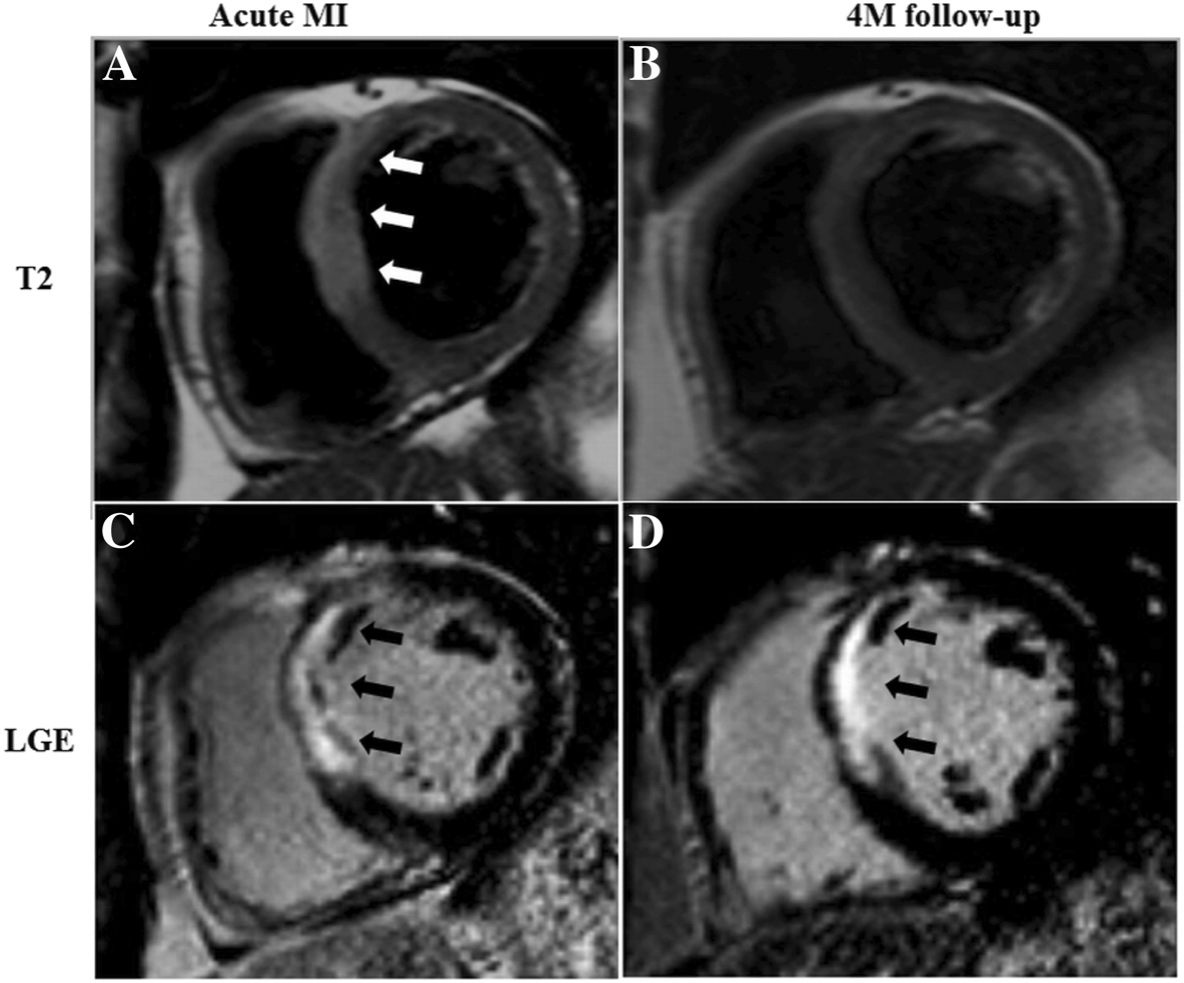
Patient example with anterior myocardial infarction (MI). Top row shows edema in the anterior/anteroseptal segments (white arrows) on T2-weighted (T2) short-axis images (equatorial slice) after acute MI (A) and absent edema at 4 months (B). Bottom row shows corresponding late gadolinium enhancement (LGE) images with scar formation in the anterior/anteroseptal segments (black arrows) after acute MI (C) and at 4 months (D).
